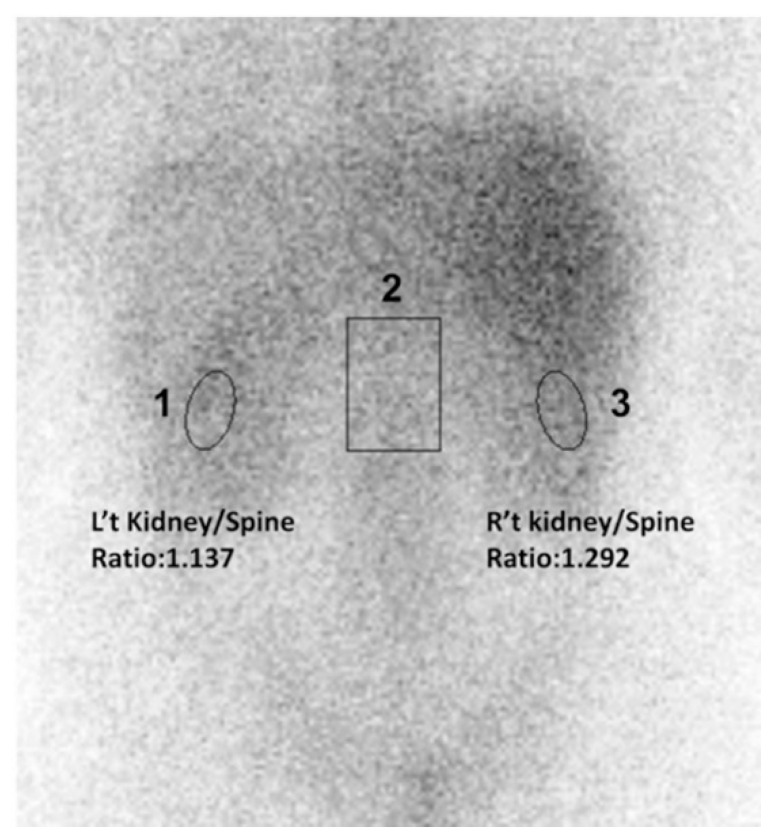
Semiquantitative measurement calculated by kidney/spine ratio from 67Ga renal image (posterior view) in a patient with lupus nephritis. ROIs on scintigraphy image: ROI 1: left kidney; ROI 2: spine; ROI 3: Right kidney. ROI: region of interest.
